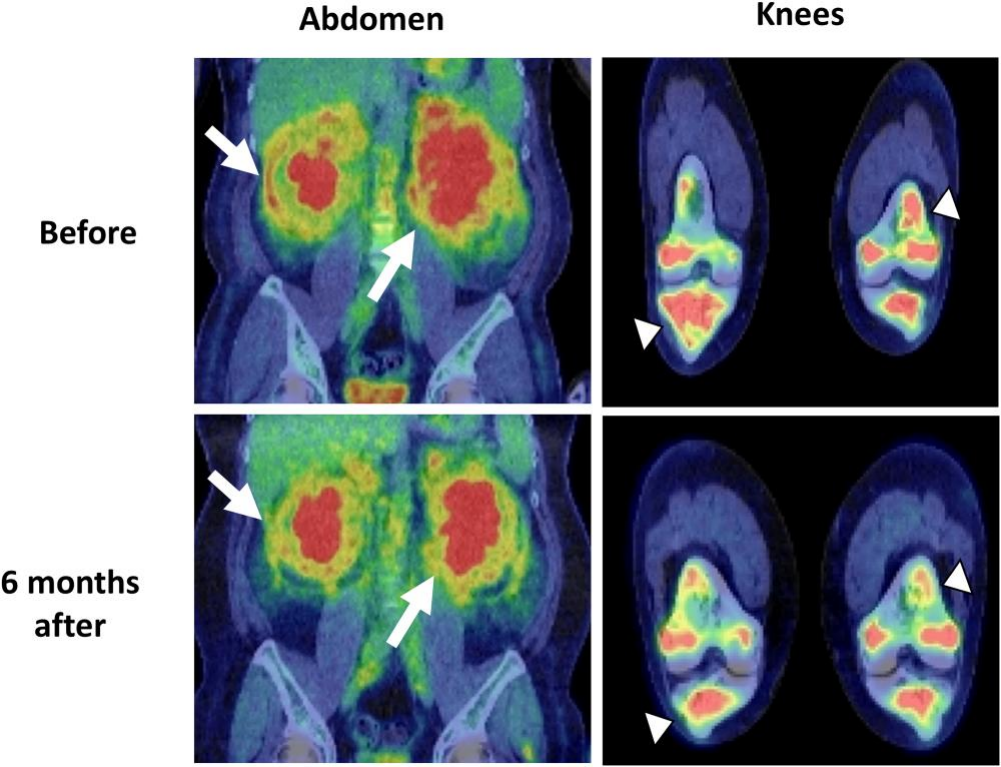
^18^F-fluorodeoxyglucose (FDG)-positron emission tomography (PET) before and 6 months after treatment. Improvement of SUV max in the abdominal lesions (from 4.8 to 3.8) (arrow) and knees (from 9.68 to 7.20) (arrowhead) can be seen.
